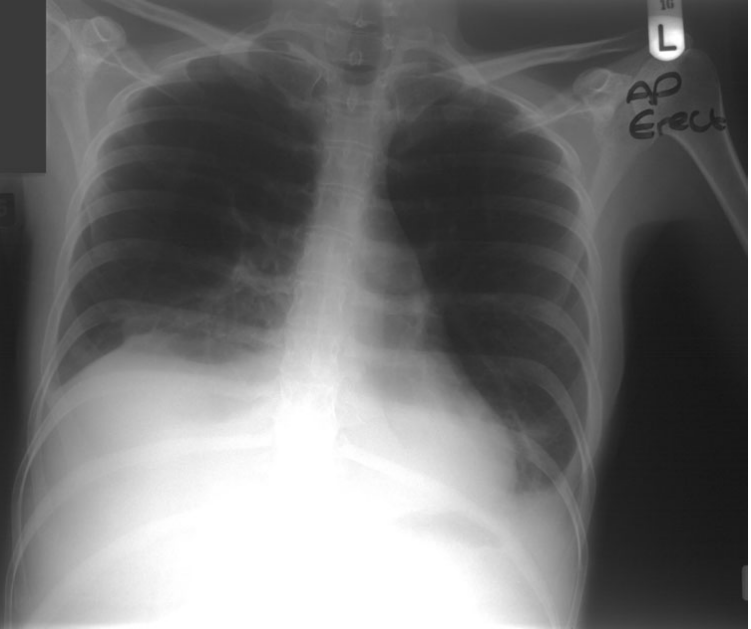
Chest radiograph demonstrating no obvious abnormality
